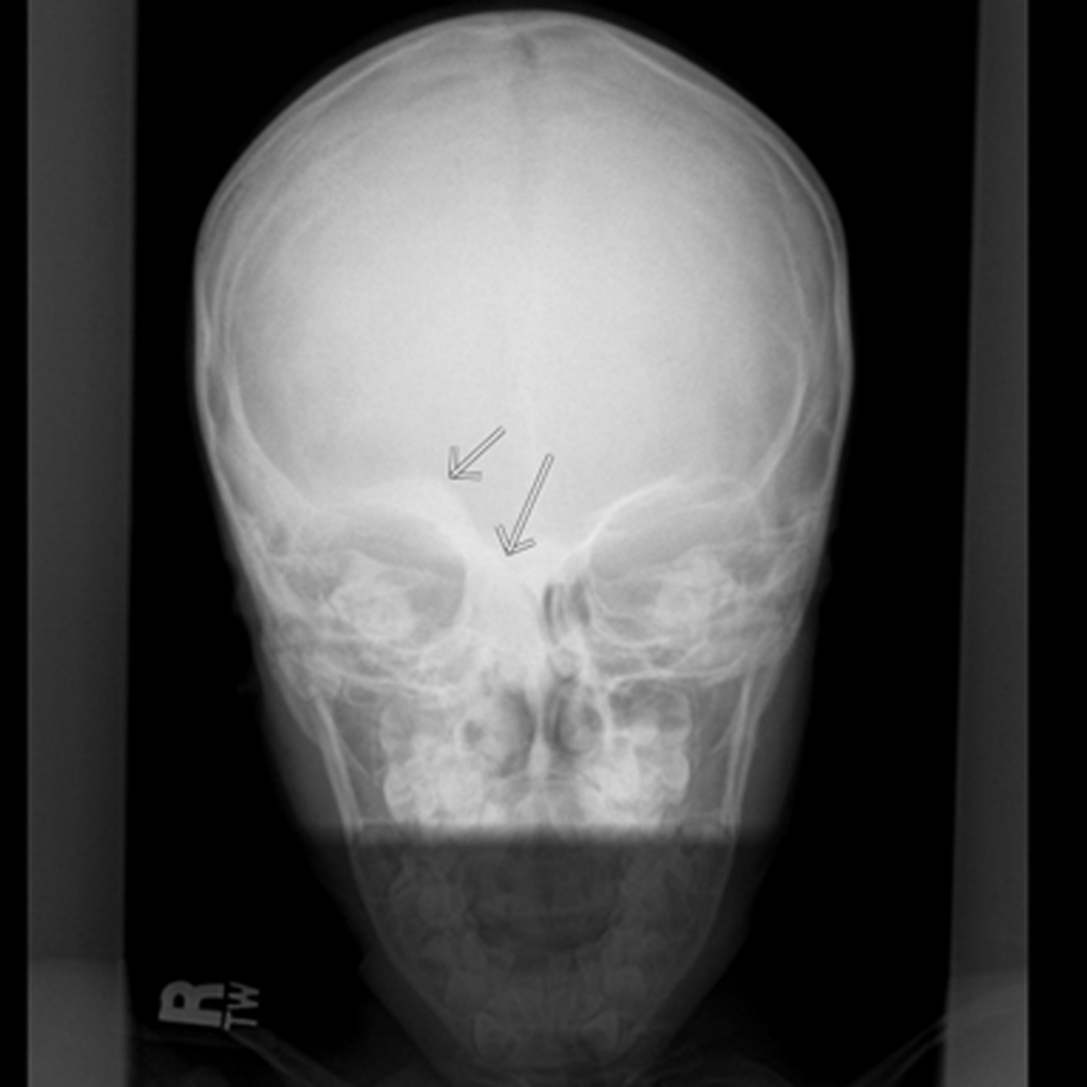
Skull X-ray of the same patient at five years of age with sclerotic lesion on right orbital rim as indicated by arrows.
